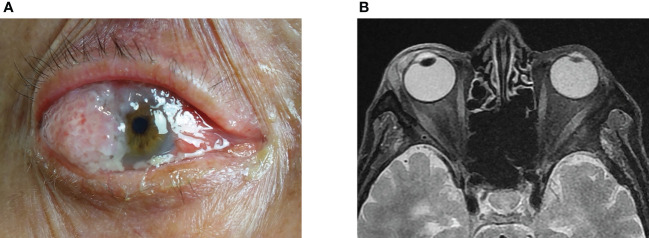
Clinical features of conjunctiva SCC showed a red, raised papilliform lesion on the conjunctiva that extended onto the cornea, with accompanying sticky mucoid discharge (A). The orbital magnetic resonance imaging demonstrated a flat tumor in front of the eyeball (B). SCC, squamous cell carcinoma.
